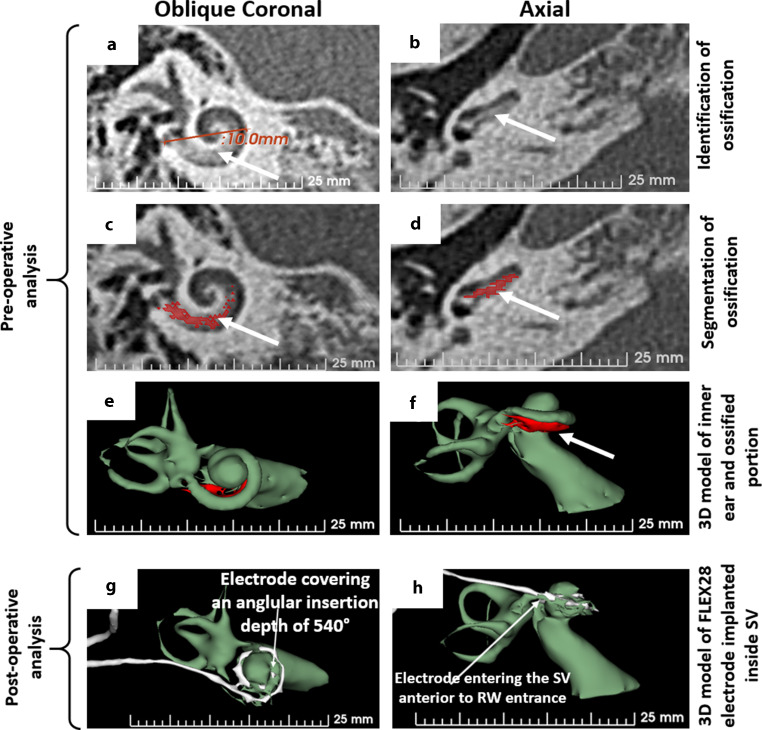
Visualization of the right ear in both oblique coronal and axial planes. Oblique coronal view of the cochlea with the basal turn diameter measured 10 mm and the white arrow pointing to basal ossification (a). Axial view showing the ossification in the basal portion of the ST (b). Segmentation of the ossified portion of ST (red shaded area) as seen in both coronal (c) and axial planes (d). 3D model (3D slicer) of the inner ear and ossification (red portion) as seen in both coronal (e) and axial planes (f). Analysis of the postoperative CT scans showed the 28 mm electrode array inserted to an AID of 540° (g) and the electrode fully placed in the SV (h)
